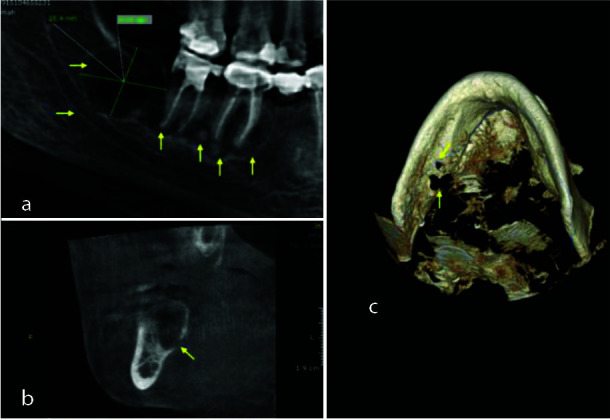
a: panoramic view of the cone beam computed tomography showing the cystic lesion measurement and cemento-osseous dysplasia lesions related to the apices of the teeth, b: Paraxial image of the cystic lesion
